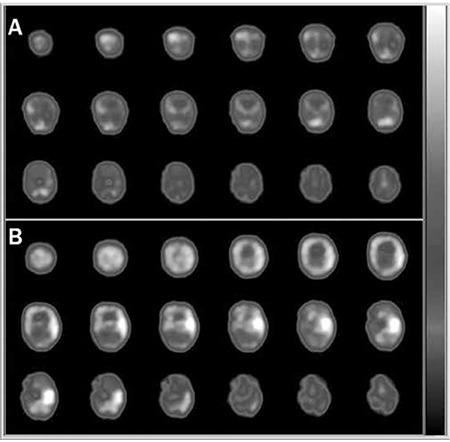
Interictal (A) and ictal (B) Tc-99m hexamethyl propylene amine oxime brain perfusion single-photon emission computed tomography (SPECT) studies of a 12-d-old infant with intractable seizures. The interictal SPECT showed an area of hypoperfusion in the left temporal cortex, whereas the ictal SPECT revealed prominent hyperperfusion in the same area. Additionally, in the interictal brain perfusion SPECT, higher tracer uptake in the primary sensorimotor cortex, thalamus, and cerebellum was observed that is typical for the neonatal period (24,25)
